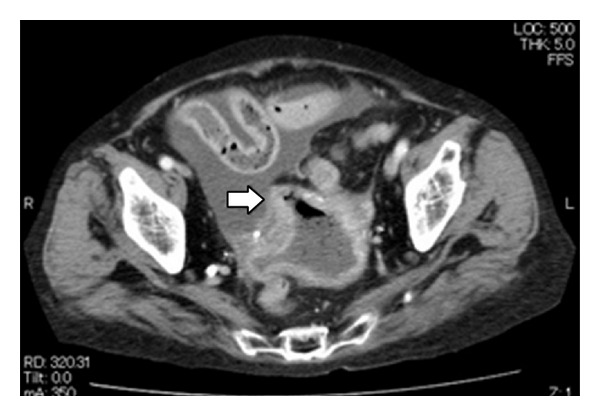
Transverse view of contrast-enhanced computed tomography scan showing the presence of fluid within the abdominal cavity and a significantly distended fluid-filled uterus. In addition, free air was detected in the abdominal and uterine cavities. White arrow indicates the perforation site.
